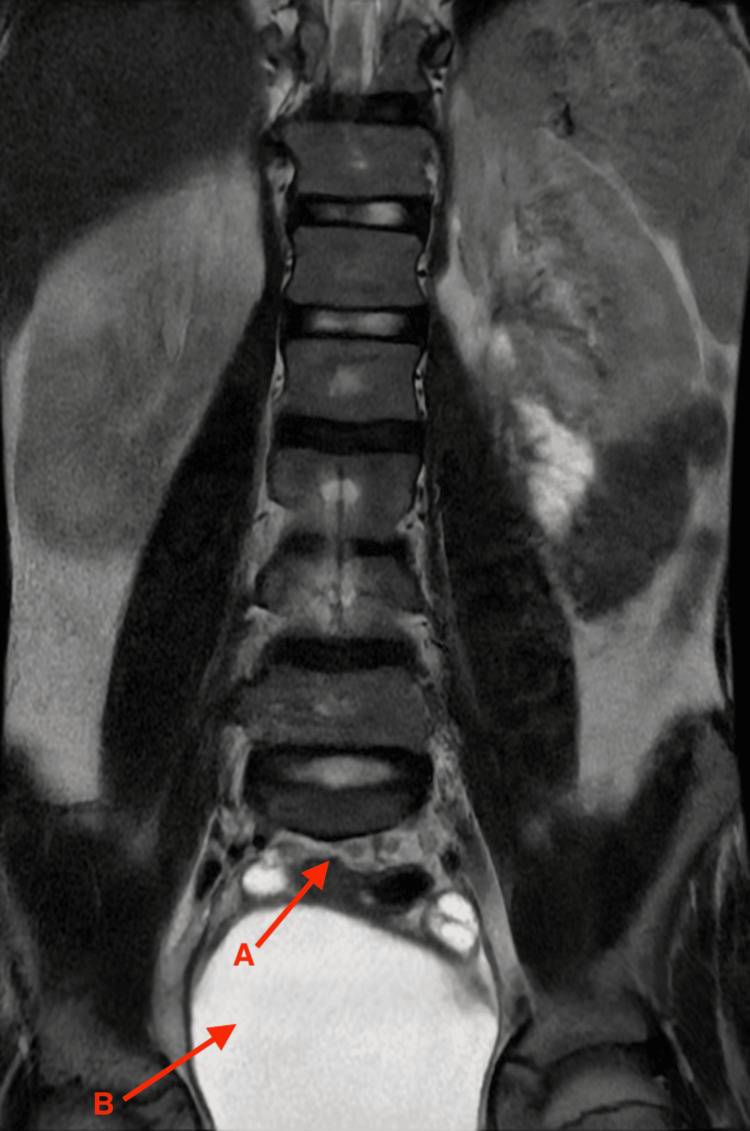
Coronal lumbar magnetic resonance imaging A: Midline bony defect with protrusion of hyperintense lipomatous tissue in continuity with the spinal canal. B: Extension of lipomatous tissue into the subcutaneous tissue, characteristic of lipomyelomeningocele.
